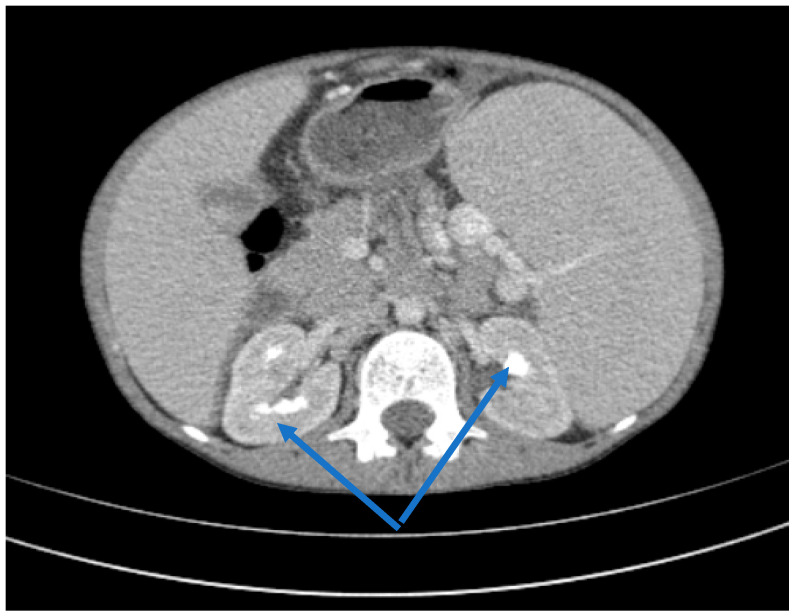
Axial CT scans, portal hypertension (blue arrow).
